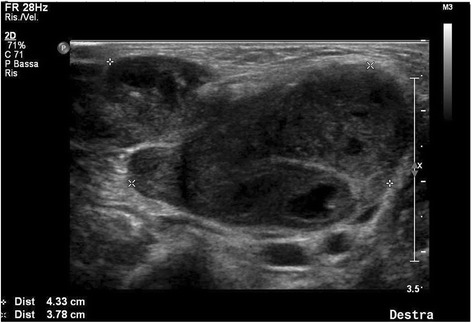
Preoperative ultrasound of patient’s lymph node
